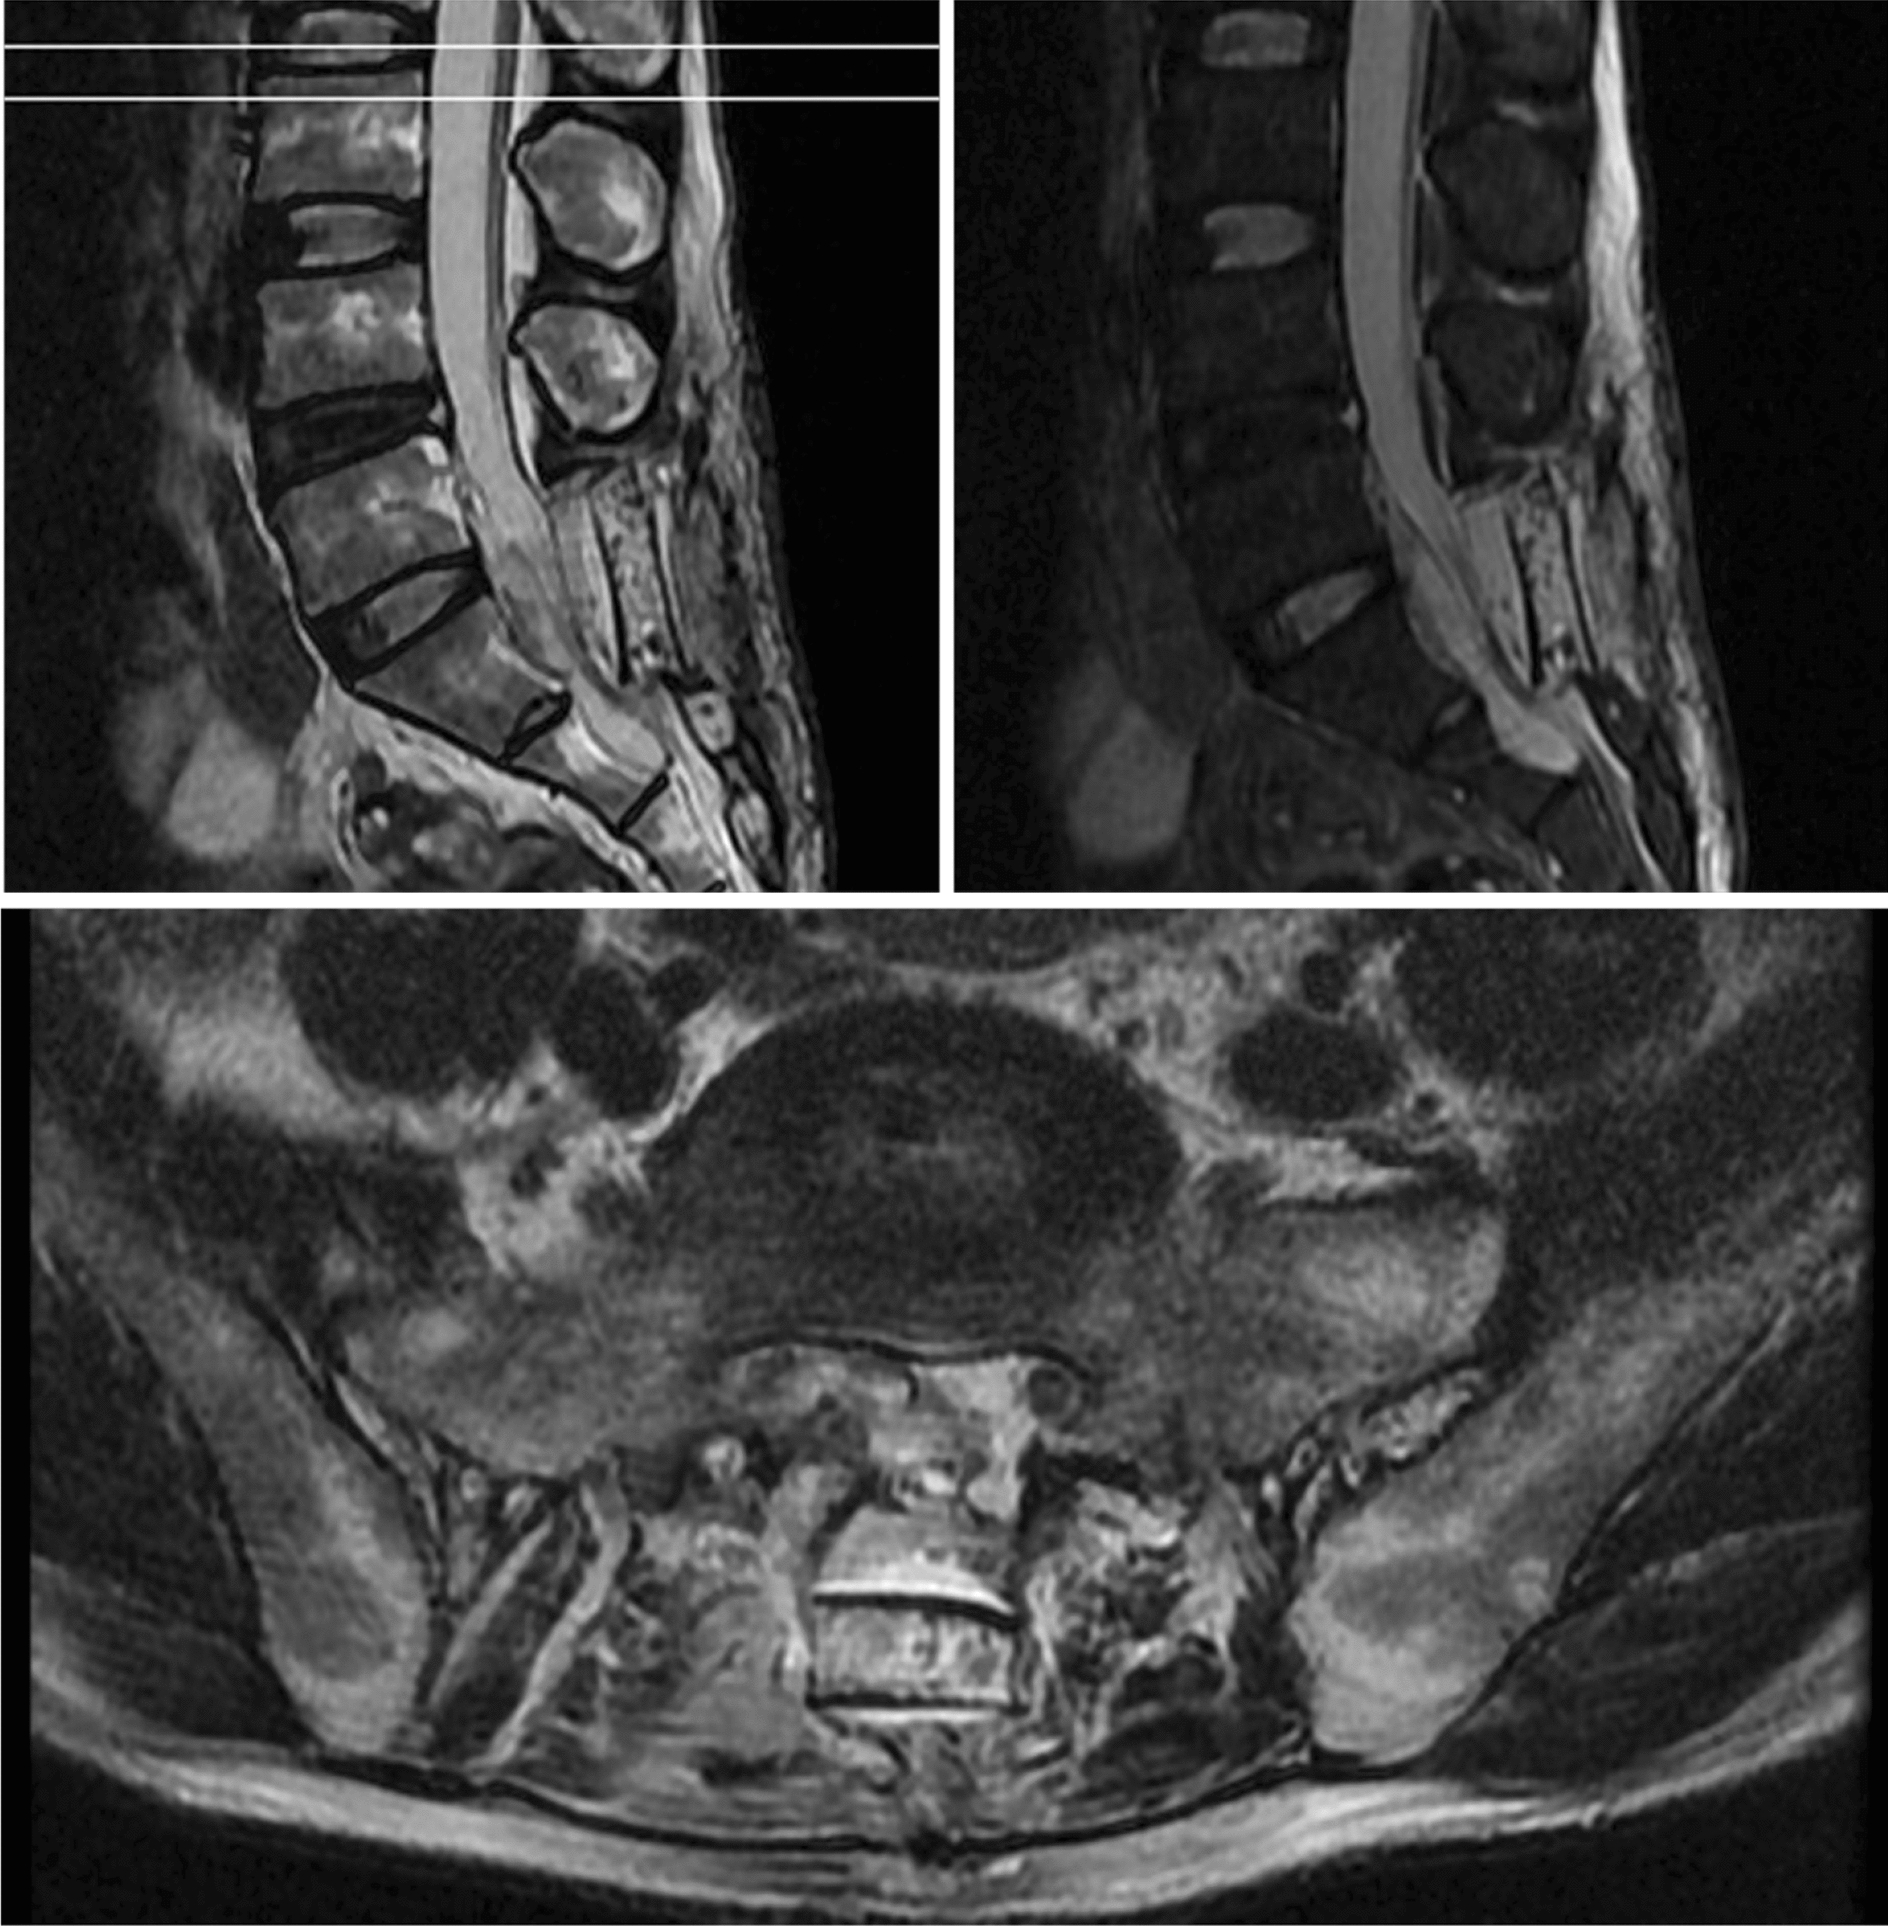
Postoperative lumbar spine MRI. S1–2 spinous process (see fixator shadow), presenting postoperative changes
